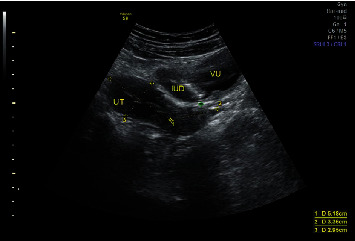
The abdominal ultrasound findings showed that all parts of the IUCD were located intravesically. UT: uterus; IUD: intrauterine contraceptive device; VU: vesica urinaria.
